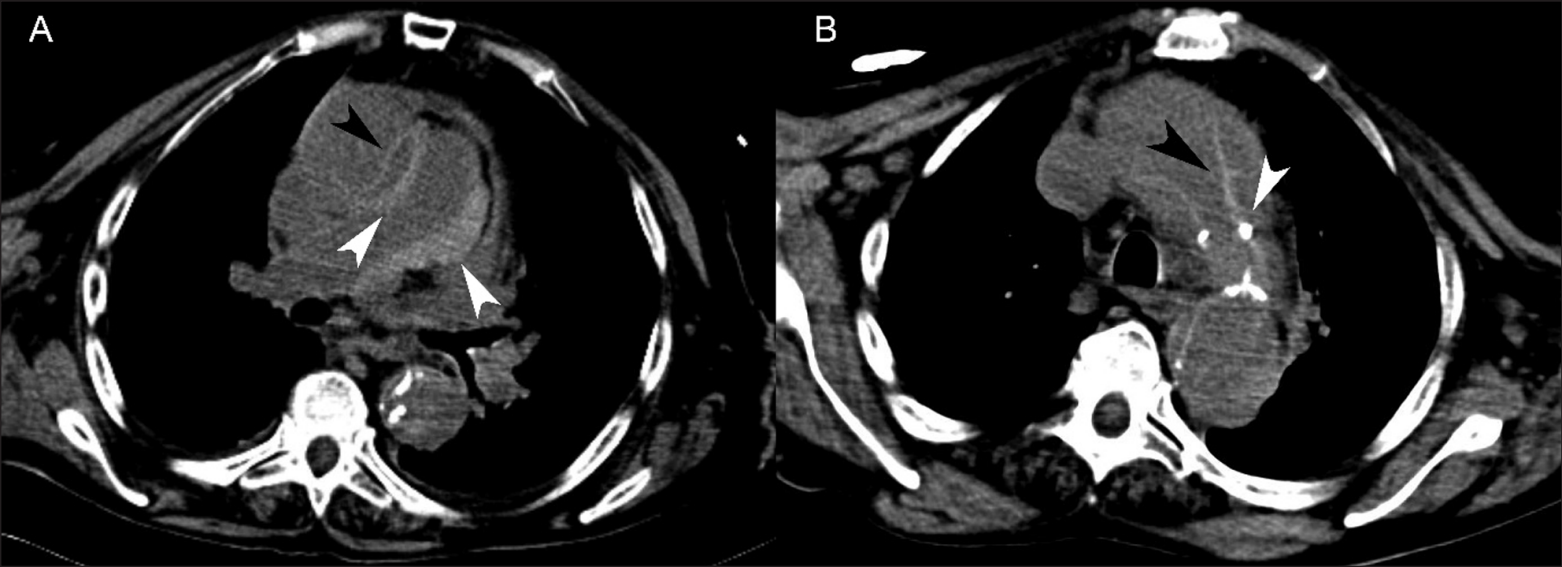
Patient 3. (A) Non-contrast-enhanced CT showed circumferential high attenuation along the walls of the main and right pulmonary arteries, consistent with pulmonary artery intramural hematoma (white arrowheads). Stanford type A aortic dissection with an intimal flap in the ascending aorta was also seen (black arrowhead). (B) Non-contrast-enhanced CT revealed an intimal flap of the thoracic aorta (black arrowhead) and displacement of atherosclerotic calcifications into the aortic lumen (white arrowhead).
